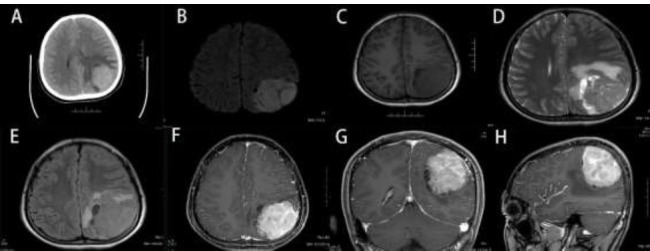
Preoperative CT and MRI demonstrate a large contrast-enhancing tumor in the left parietal lobe. (A) Axial CT. (B) Diffusion-weighted imaging axial MRI. (C) T1-weighted axial MRl. (D) T2-weighted non-contrast axial MRl. (E) Fluid Attenuated Inversion Recovery (FLAIR)-weighted axial MRI. (F) T1-weighted contrast-enhanced axial MRI. (G) T1-weighted contrast-enhanced coronary MRI. (H) T1-weighted contrast-enhanced sagittal MRI.
